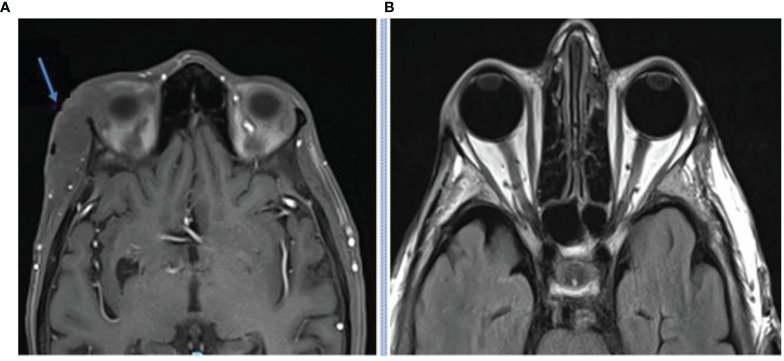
MRI imaging at baseline (A) and after 5 months of cemiplimab 350 mg/3-weekly (B) demonstrating resolution of the soft tissue orbital mass.
